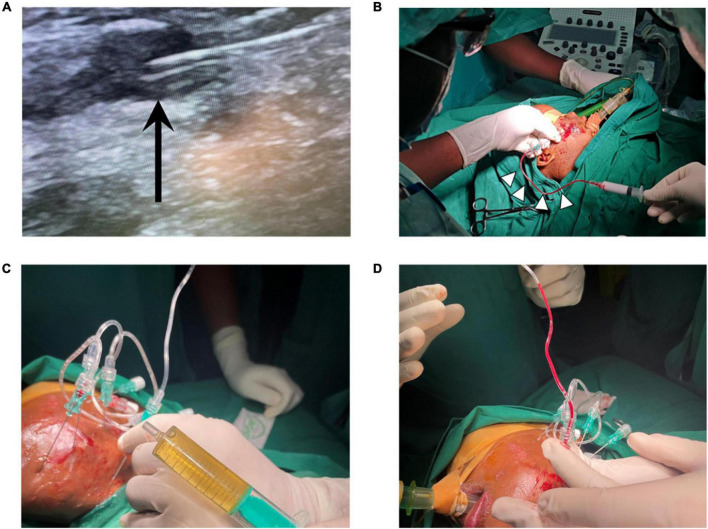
(A) Ultrasound-guided needle placement in dysplastic veins of a VM, black arrow points at needle tip. (B) Aspiration of blood (white arrow heads) confirms needle position within dysplastic VM vessels prior to sclerotherapy with polidocanol foam. (C) Aspiration of lymph fluid and (D) aspiration of blood in a mixed-type lymphatic and venous malformation, treatment with bleomycin (for lymphatic cysts) and polidocanol (for venous components). VM = venous malformation.
